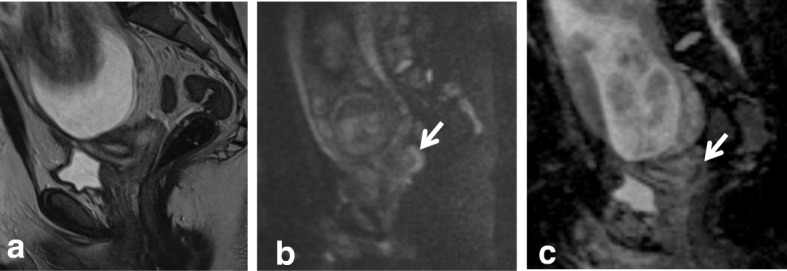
Pregnant woman with biopsy-confirmed cervical cancer that is not detectable on T2WI (a), but is delineated (white arrows) on DWI images (b, c)
